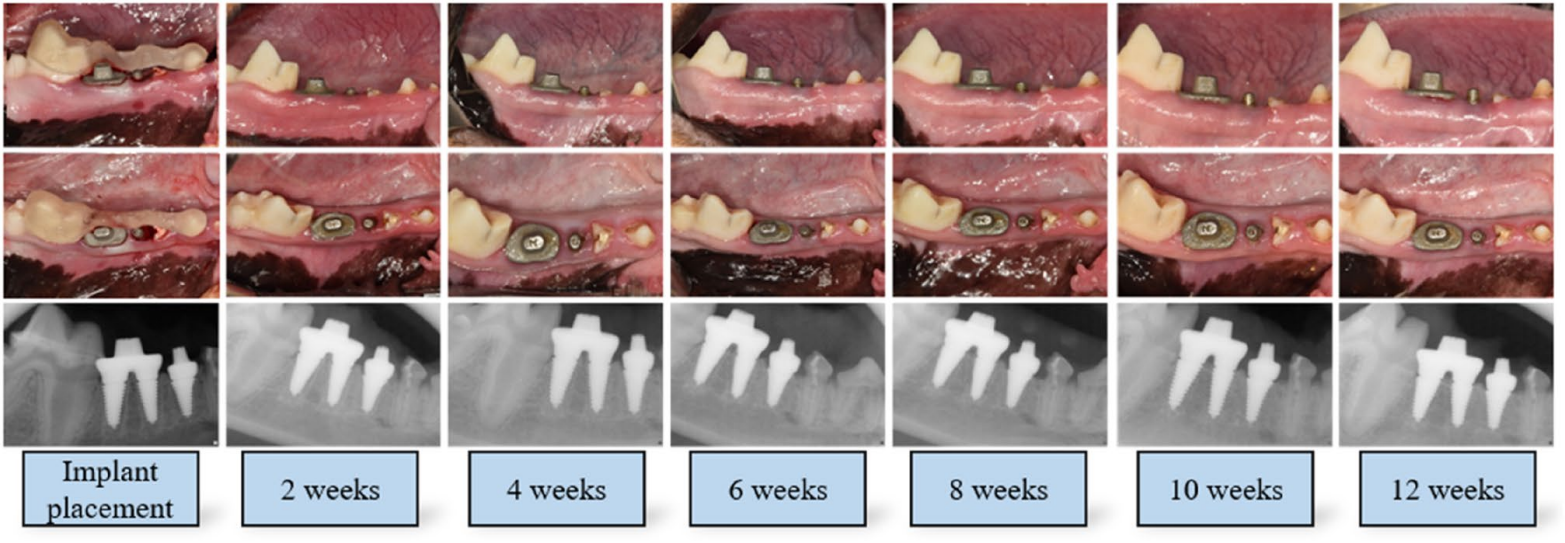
Clinical and radiographic photograph of single- and double-root 3D-printed implant. All protective caps were removed 2 weeks after implant placement for plaque control and implant stability measurements. There were no clinical signs of peri-implant inflammation, including redness, spontaneous bleeding, swelling, or ulceration. The clinical and radiographic photos were taken at the time of implant placement and 2, 4, 6, 8, 10, 12 weeks after implant placement.
